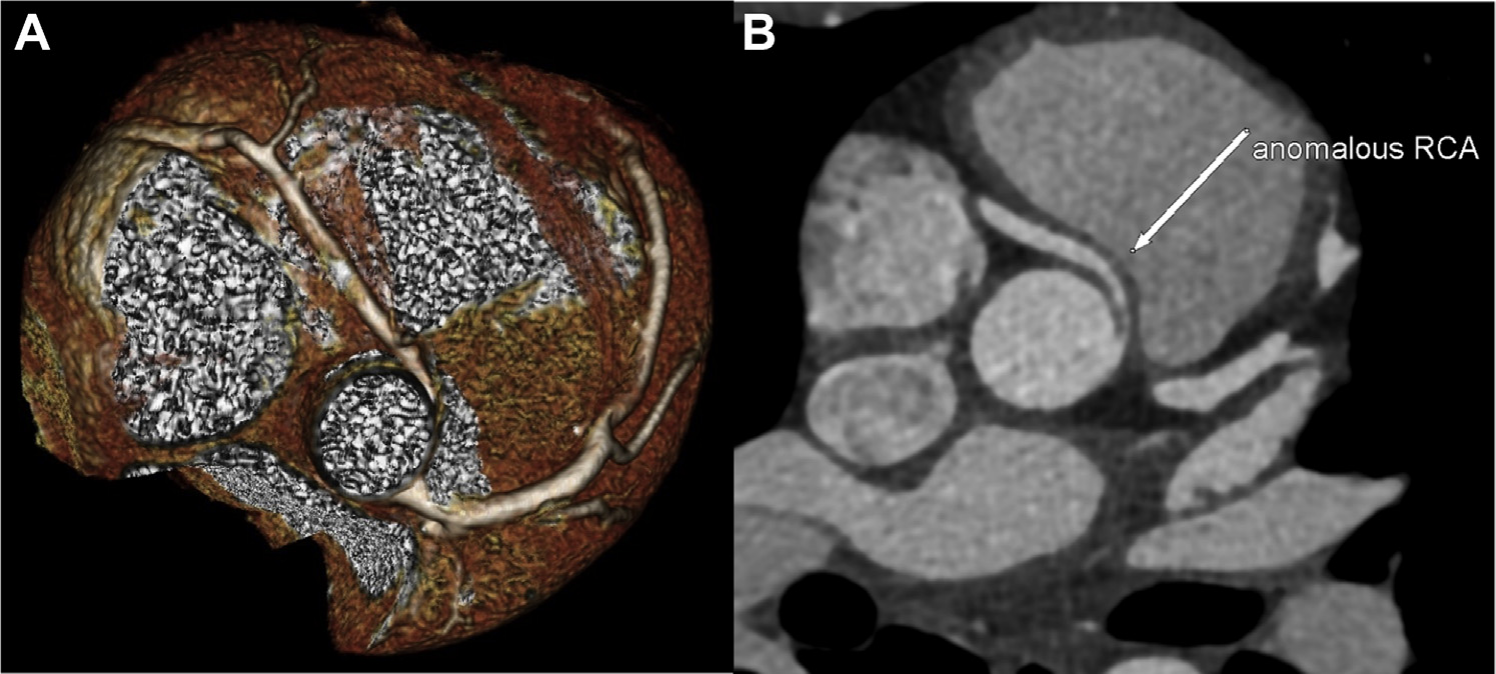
Computed Tomography Coronary Angiography of the Anomalous Right Coronary Artery (A) 3-dimensional volume-rendered and (B) axial multiplanar reformat images obtained from computed tomography coronary angiography of the anomalous right coronary artery, which follows a malignant interarterial course between the aorta and the pulmonary artery.
